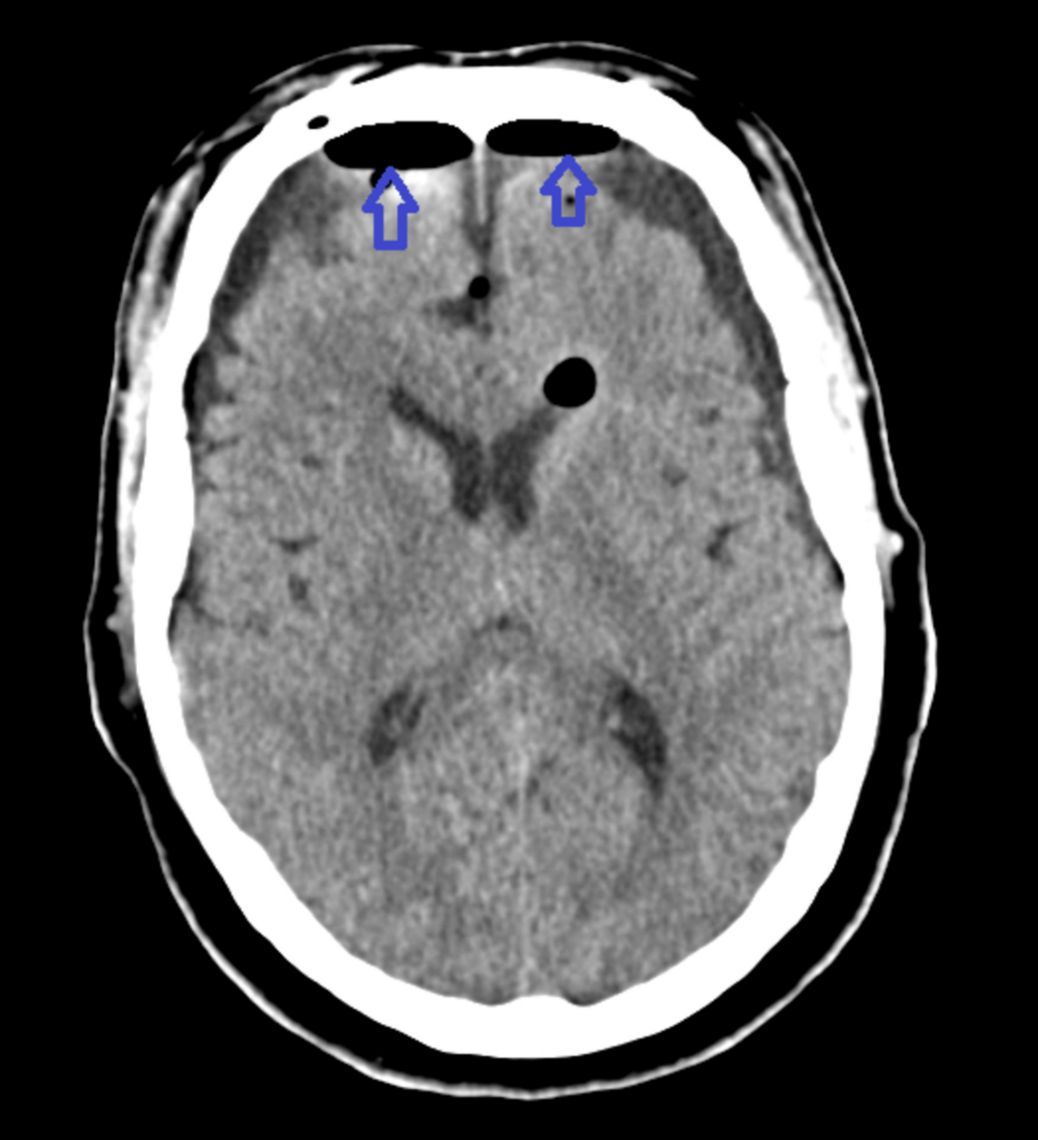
Computed tomography of the head showing significant improvement in tension pneumocephalus post-procedure The blue-colored arrowheads point towards small bifrontal collections, likely hygromas.
